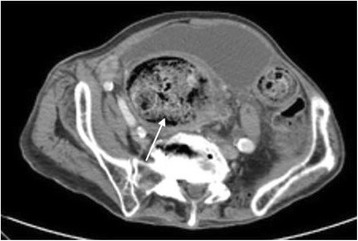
CT scan image of the abdomen showing large amount of retained stool in the colon with bowel wall thickening and fat stranding
